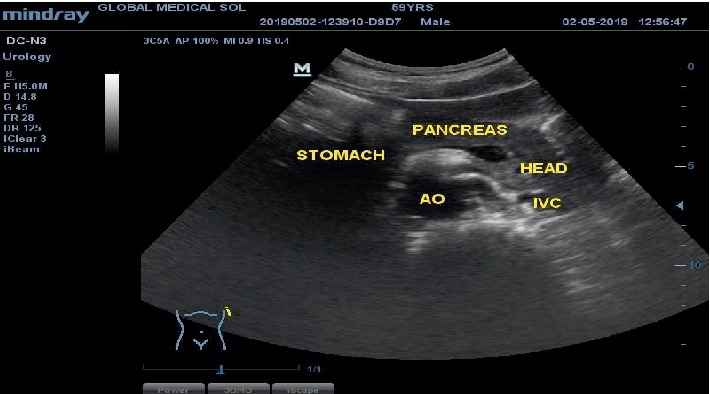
Transverse sonogram without Colour Doppler of the epigastrium. The head of the pancreas on the left side and a gas filled stomach on the right side. Abdominal aorta (AO) on the right side and inferior vena cava (IVC) on the left.
